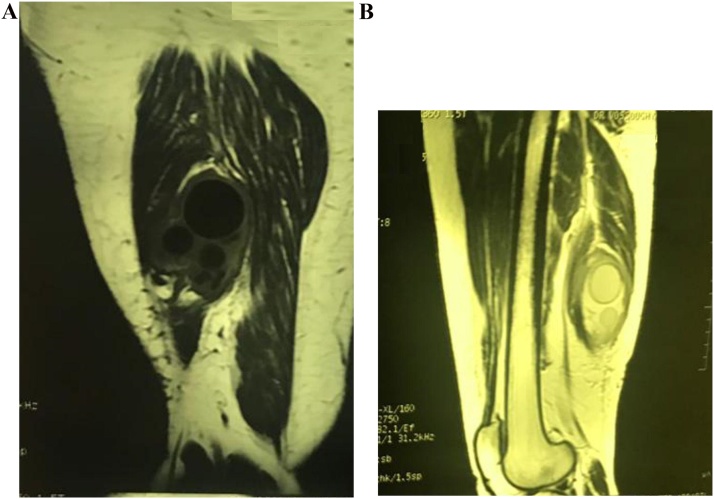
MRI showing cystic swelling in biceps femoris in T1 (a) and T2 (b) view.
